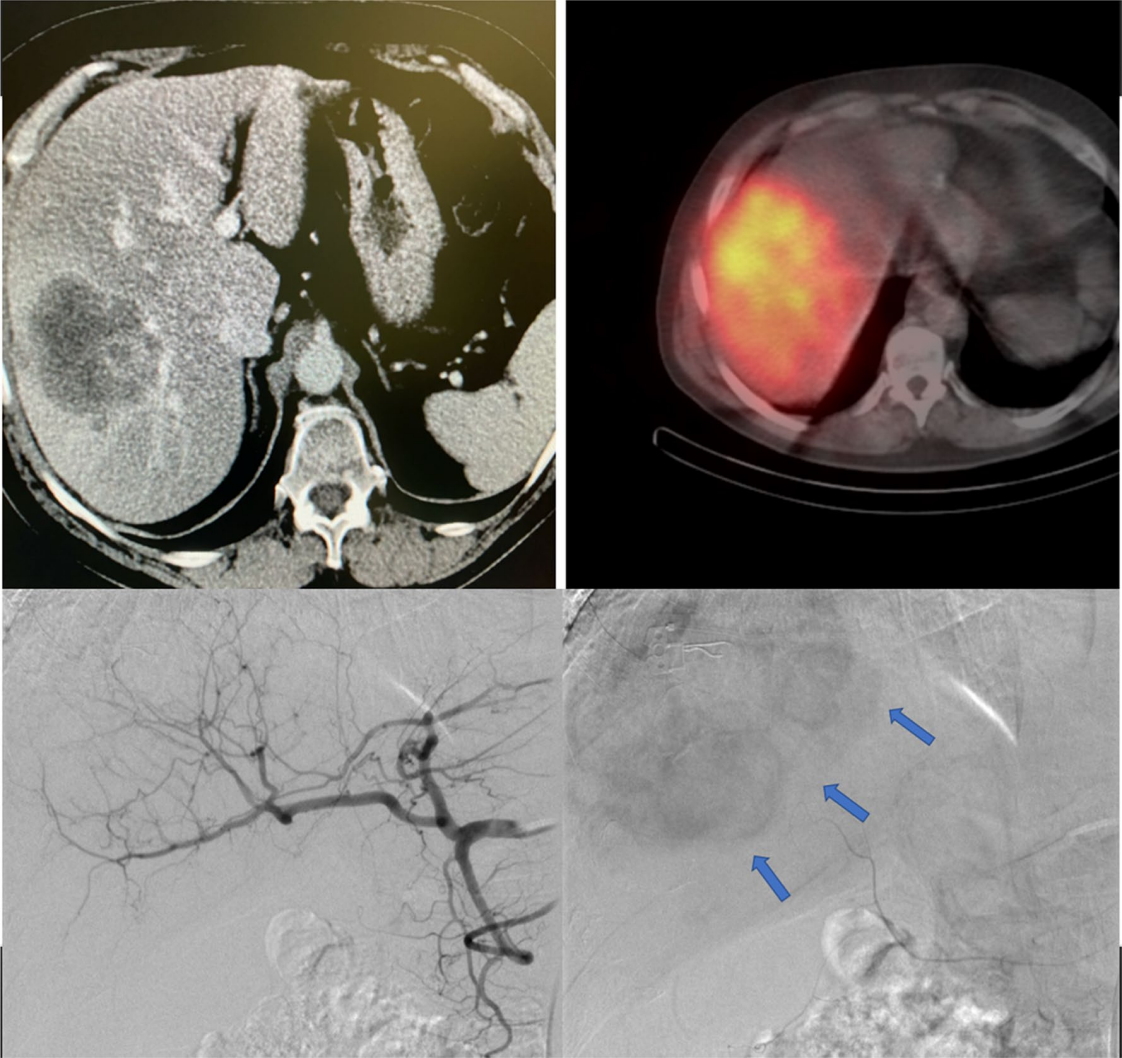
Non-homogenous areas in a solid liver tumor (mCRC) in contrast-enhanced computed tomography and non-homogenous spread of beta-emitting microspheres within a tumor in SPECT/CT (A,B). Angiogram of hepatic arteries and hepatic tumors contrast enhancement—blue arrows (C,D). Images of one of the patients treated with radioembolization in our center (with permission).
